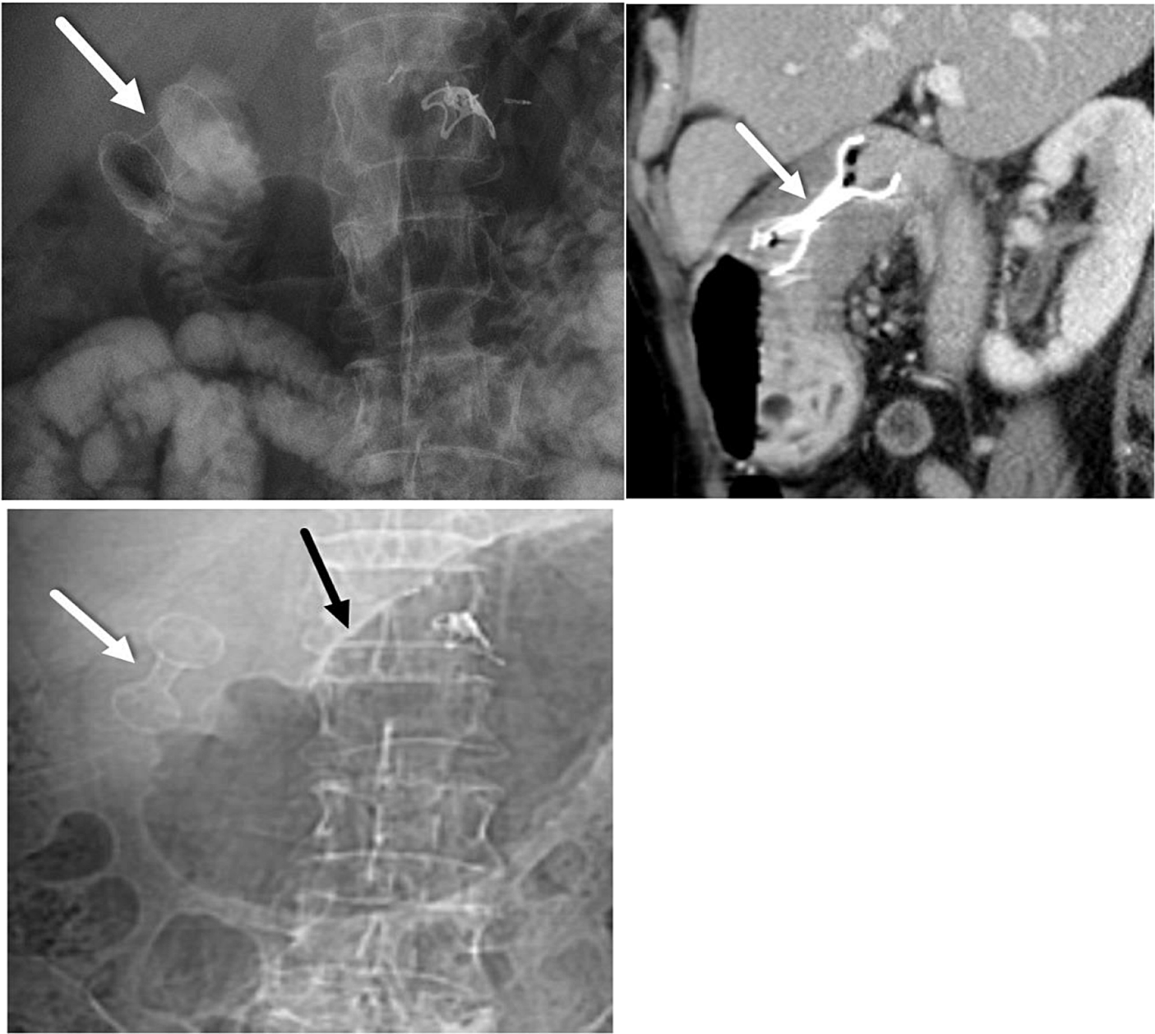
63 year-old patient presenting with benign pyloric strictures secondary to peptic ulcer disease leading to gastric outlet syndrome. A. Abdominal radiograph shows intraluminal LAMS (white arrow) that was placed to relieve a benign pyloric stricture with significant clinical improvement. B. Contrast-enhanced sagittal CT image obtained a year later, when the patient returned after being lost to follow-up, demonstrates new luminal narrowing (white arrow) due to mucosal ingrowth of LAMS placed across the pylorus. C. A frontal CT topogram obtained at the same time when the patient represented demonstrates new narrowing (white arrow) of the LAMS lumen as a complication due to mucosal ingrowth and resultant recurrent gastric outlet obstruction (dilated stomach (black arrow))
